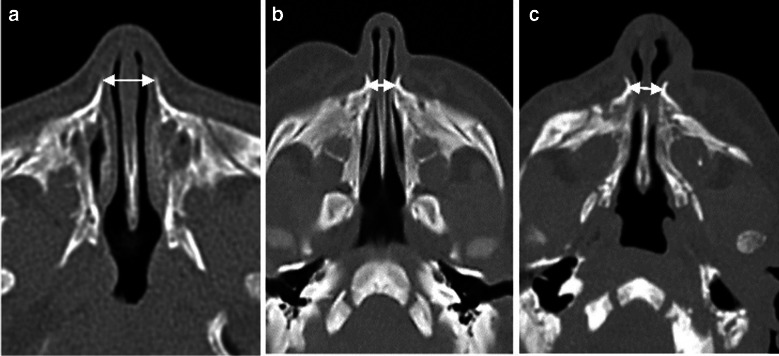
Axial computed tomography (CT) slices (without contrast) showing three examples of manual measurements of the pyriform aperture width using a bidirectional arrow. a A 4-day-old girl with normal pyriform aperture width (11 mm). b A 4-day-old girl with congenital nasal pyriform aperture stenosis treated conservatively (pyriform aperture width = 4.7 mm). c A 2-day-old boy with pyriform aperture stenosis who underwent surgery (pyriform aperture width = 6.7 mm)
